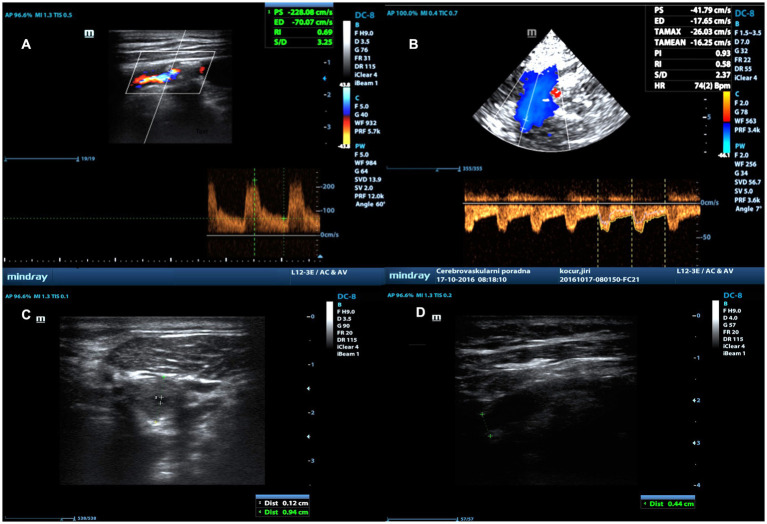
Measurement of percentage of stenosis using PSV_ICA/ICA_ ratio using duplex ultrasonography. Measurement of PSV in stenosis using linear probe (A), PSV distal to stenosis using transcranial probe (B), residual lumen in stenosis (C) and lumen diameter distal to stenosis (D) in B-Mode using linear probe.
